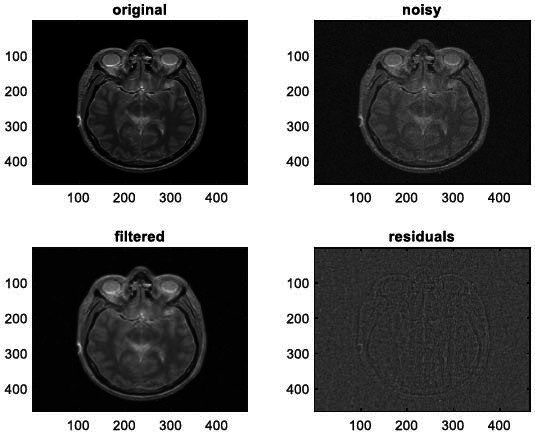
The sixth example of MRI denoising by applying the proposed image denoising technique.
